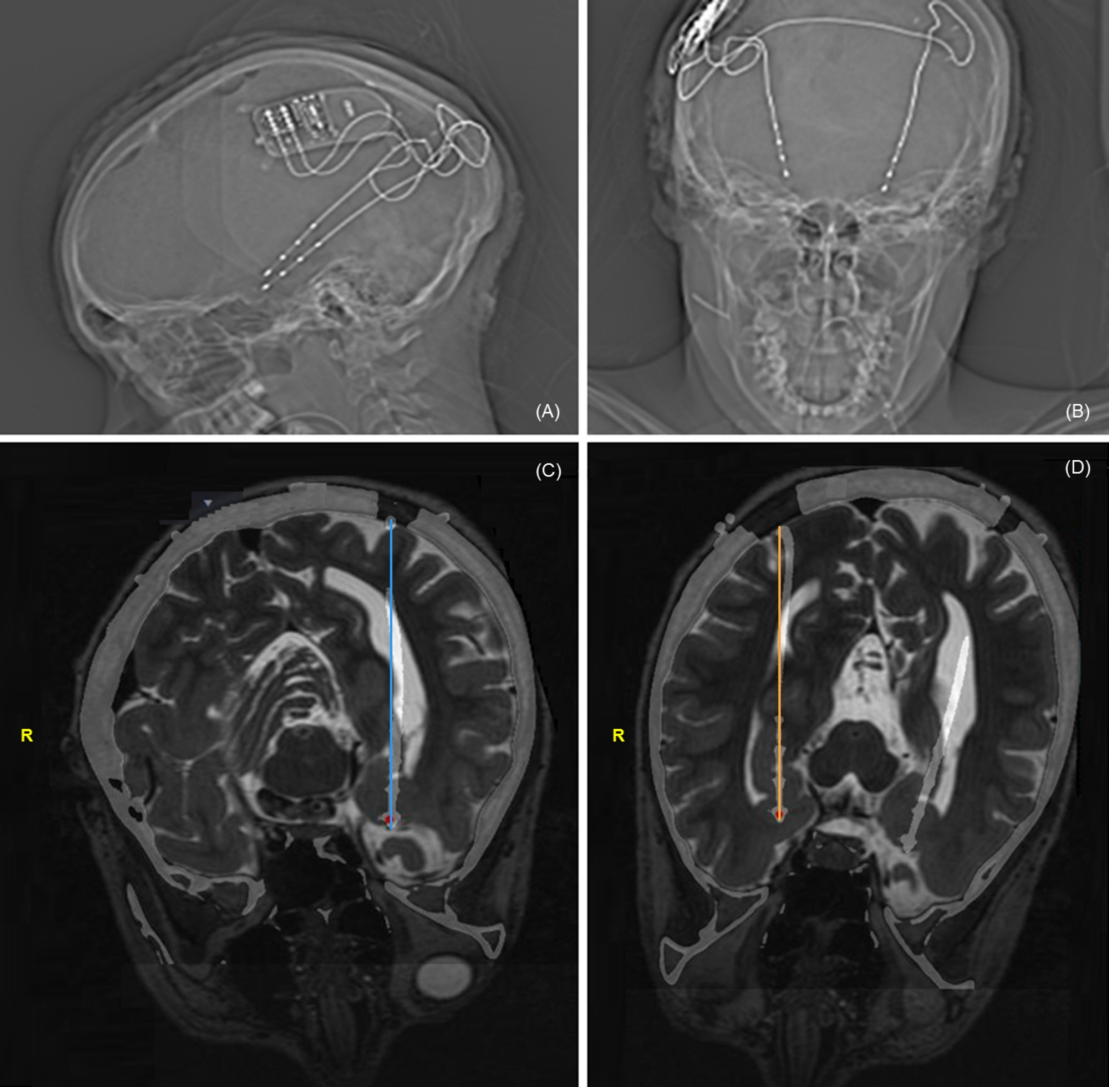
Postoperative X-rays (A-B) and merged CT-MRI (C-D) Postoperative X-rays (A-B) and merged CT-MRI (C-D) demonstrating the final lead position in bilateral mesial temporal structures (C-left, D-right) with associated curvature during intraventricular course.
